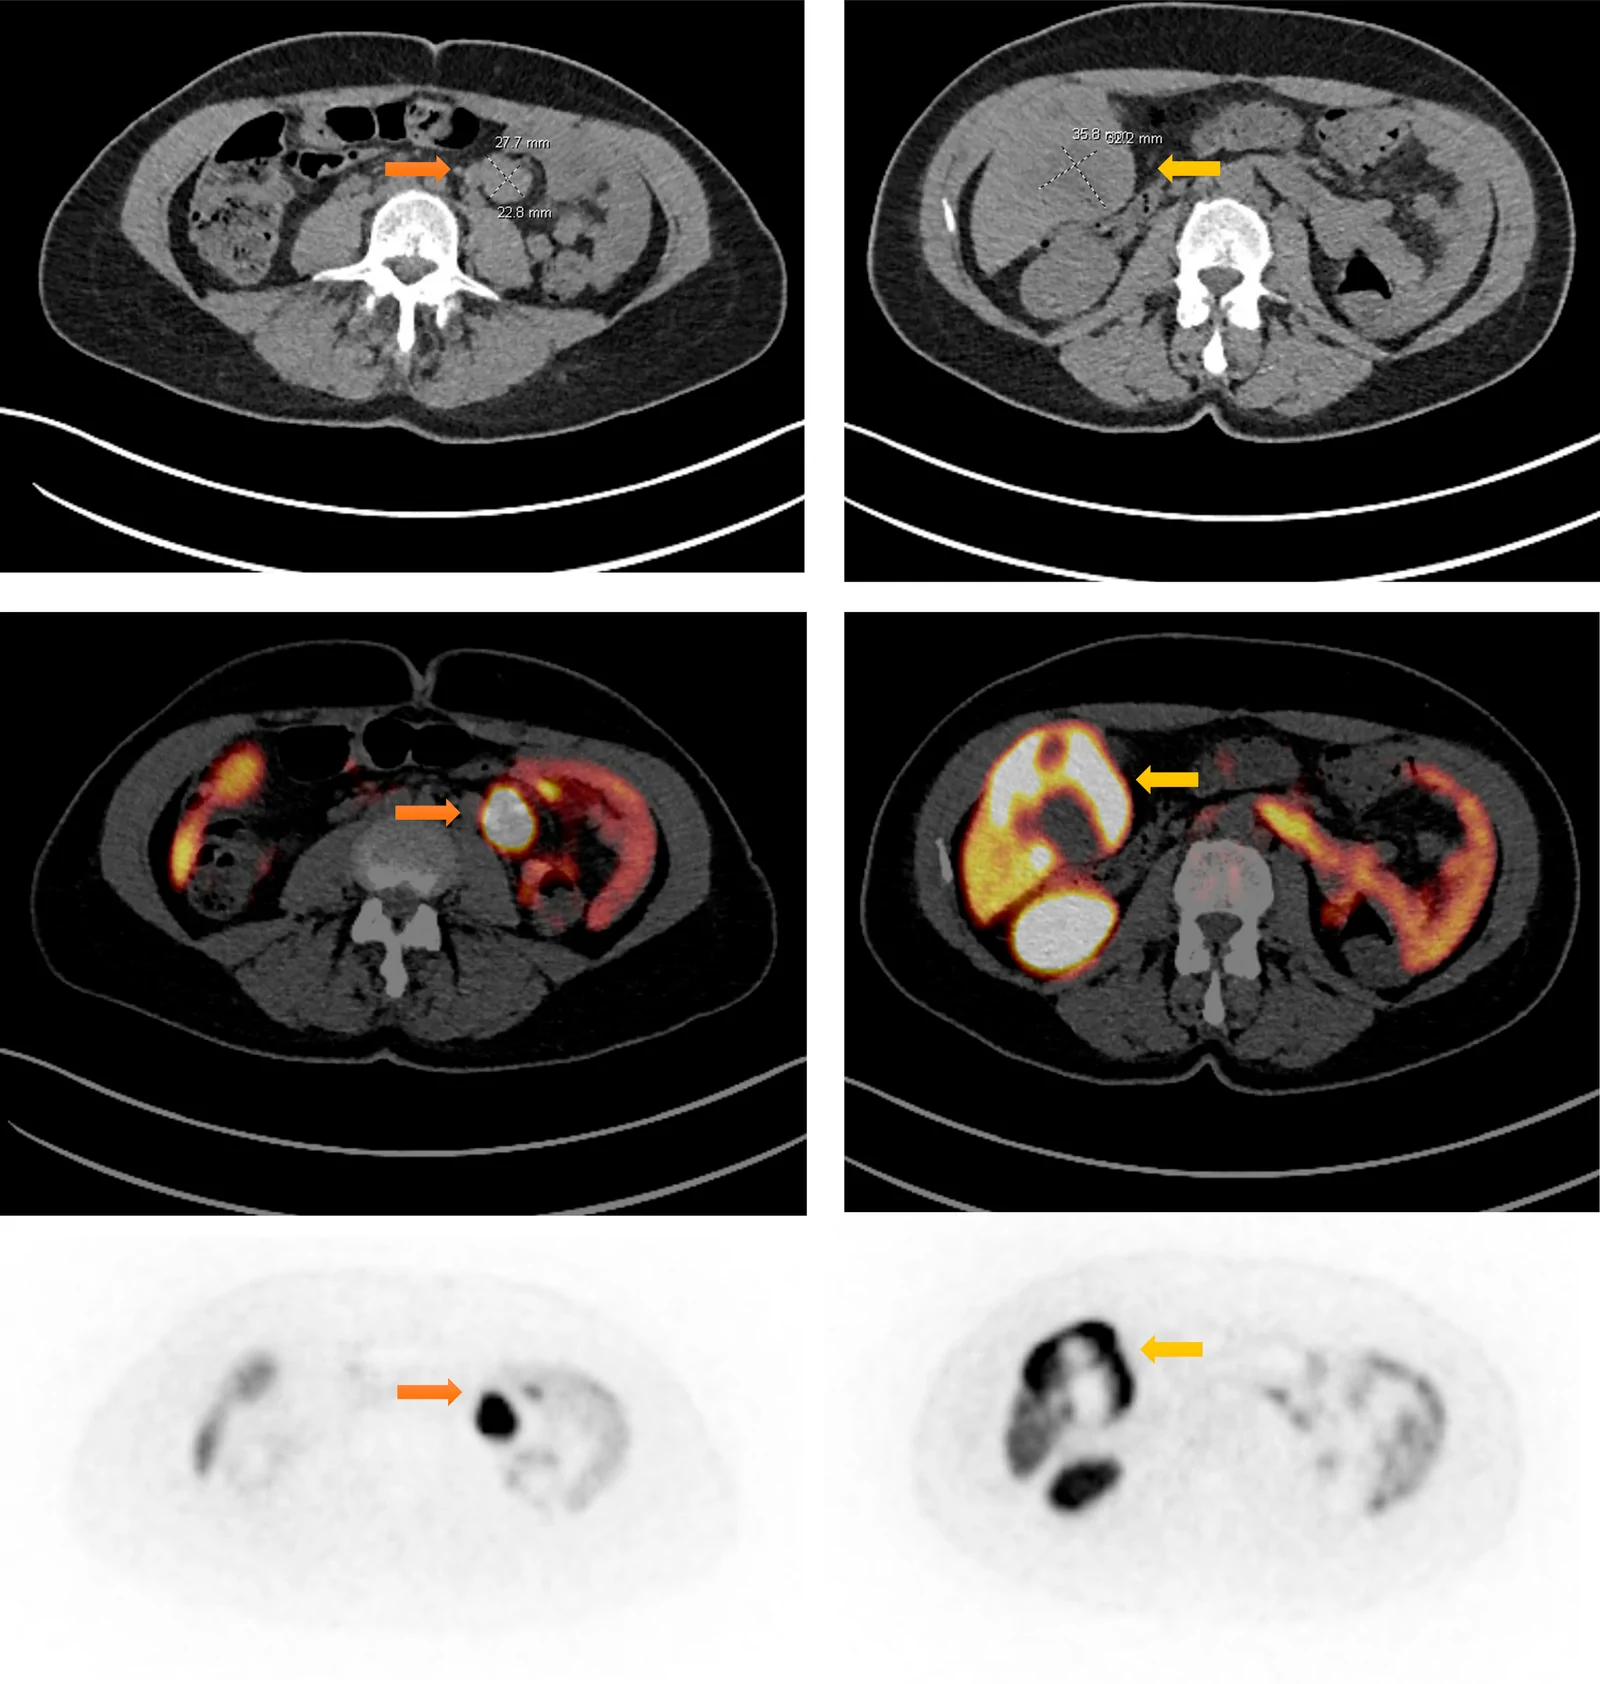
Findings on Gallium - 68 DOTATATE Positron Emission Tomography/Computed Tomography Gallium-68 DOTATATE positron emission tomography/computed tomography scan showing soft tissue mass in the left mid abdomen (red arrow) and ill-defined hypodensity in hepatic segment V (yellow arrow).
